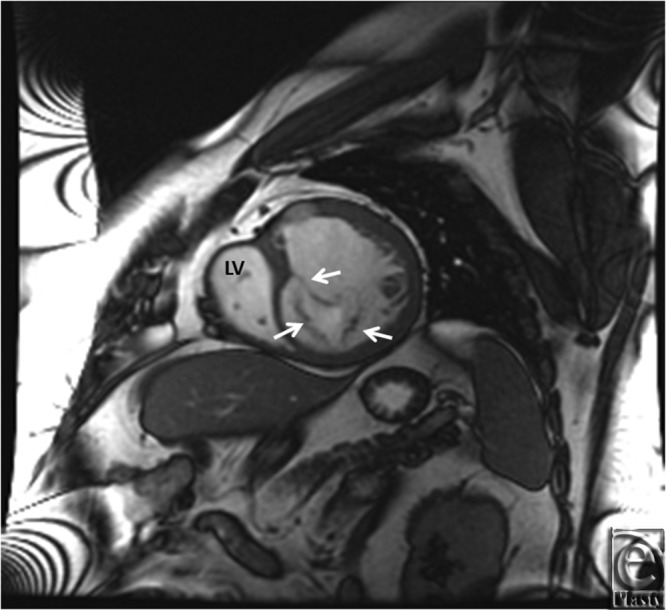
MRI SSFP showing trileaflet valve between left atrium and morphological right ventricle. LV indicates left ventricle; MRI, magnetic resonance imaging. White arrows indicate trileaflet valve.
